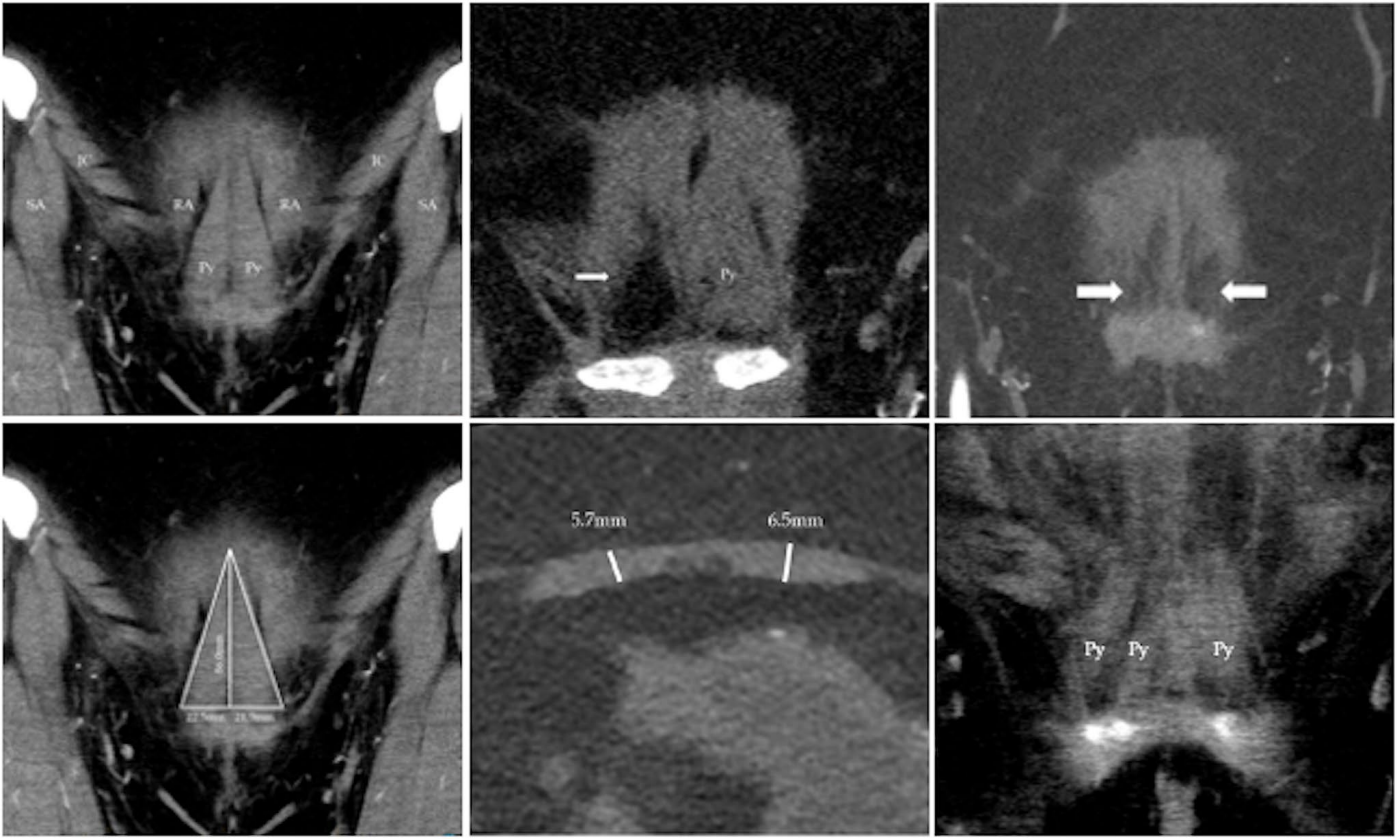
a-f CTA images. a: coronal view showing bilateral pyramidalis presence. Pyramidalis (Py), rectus abdominis (RA), sartorius (SA), inguinal canal (IC). b: coronal view showing unilateral pyramidalis presence. Pyramidalis (Py) and fatty tissue deposition in place of the right pyramidalis (white arrow). c: coronal view showing fatty tissue deposition in place of the pyramidalis, suggestive of pyramidalis absence (white arrows). d: coronal view showing measurement methodology for medial border height and base width. Pyramidalis (Py), rectus abdominis (RA), sartorius (SA), inguinal canal (IC). e: axial view showing measurement methodology for pyramidalis thickness. f: coronal view, showing pyramidalis duplicity (two pyramidalis muscles on the right and one on the left). Pyramidalis (Py)
